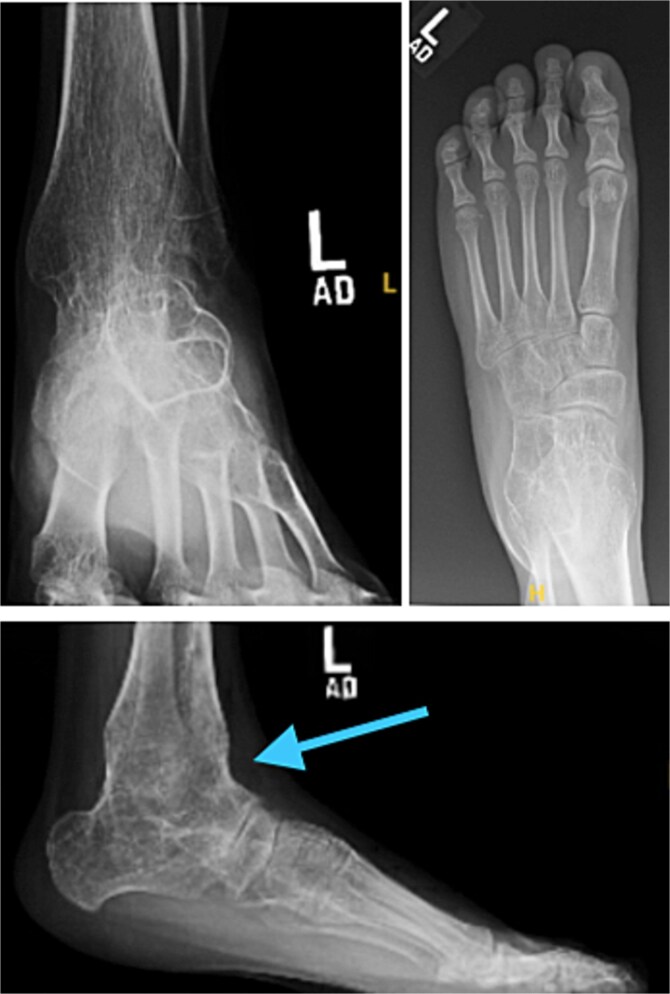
Preoperative radiographs of the left foot and ankle demonstrating congenital subtalar coalition with associated rigid equinus deformity. Anteroposterior and oblique views show hindfoot osseous fusion and altered midfoot alignment, while the lateral view (arrow) highlights the fixed plantarflexed position of the ankle and hindfoot.
